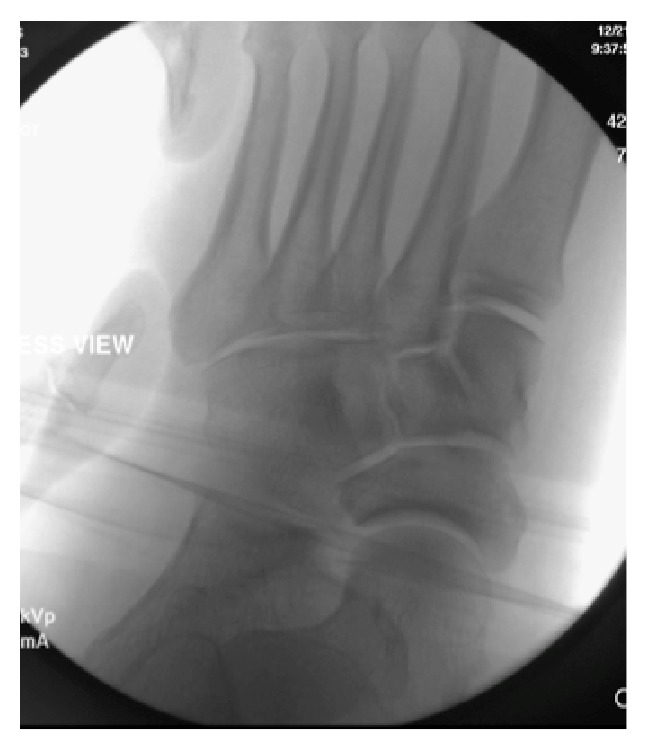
Intraoperative stress radiographs demonstrating intact first to third tarsometatarsal joints.
